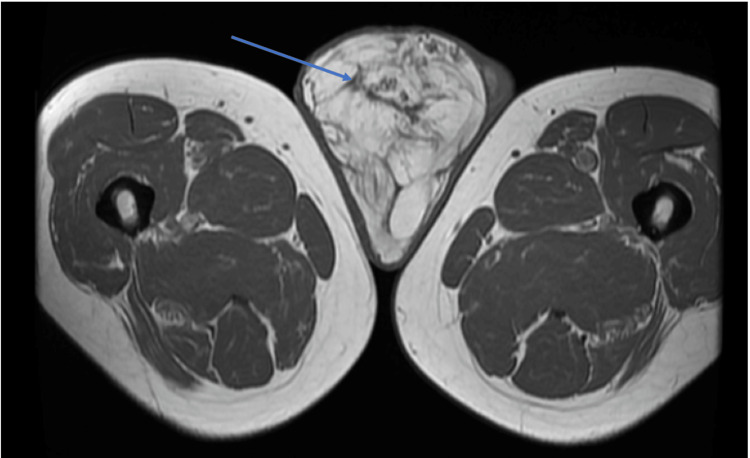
Case 2: An MRI of the pelvis and scrotum showing an extensive mass in the right hemi-scrotum of a fatty composition and enhancing septations. The tumour had grown significantly over the four months since the earlier ultrasound (Figure 3).
